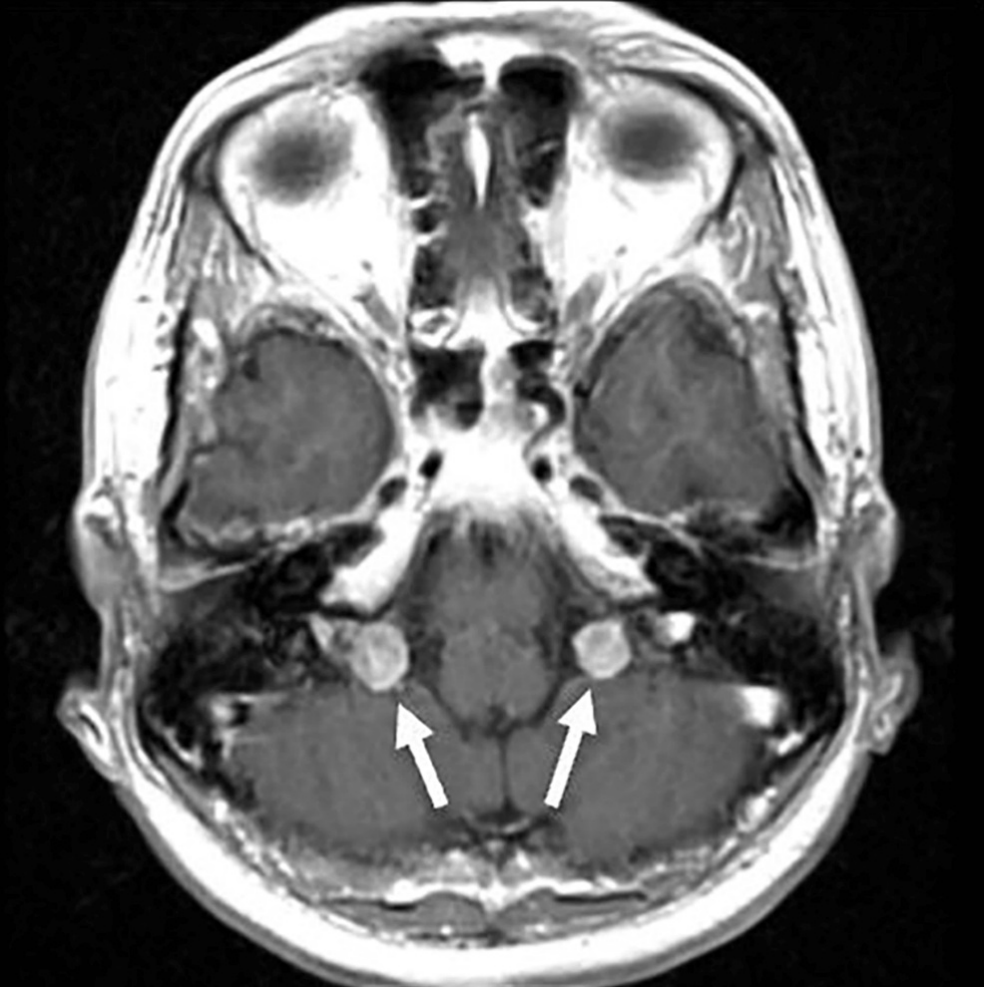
T1 MRI post-contrast demonstrating bilateral round enhancing cerebellopontine angle jugular foramen lesions (arrows), 1.1 cm on the right and 0.9 cm on the left in maximal dimension.
